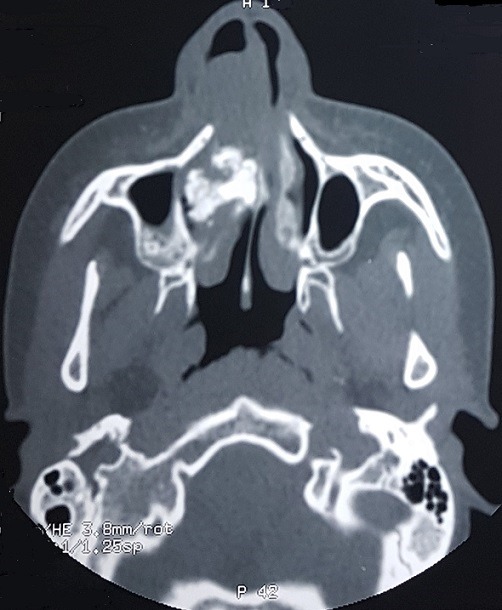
Axial sinonasal computed tomography (CT) showing rhinolite at the medium 1/3 of the right nasal fossa
